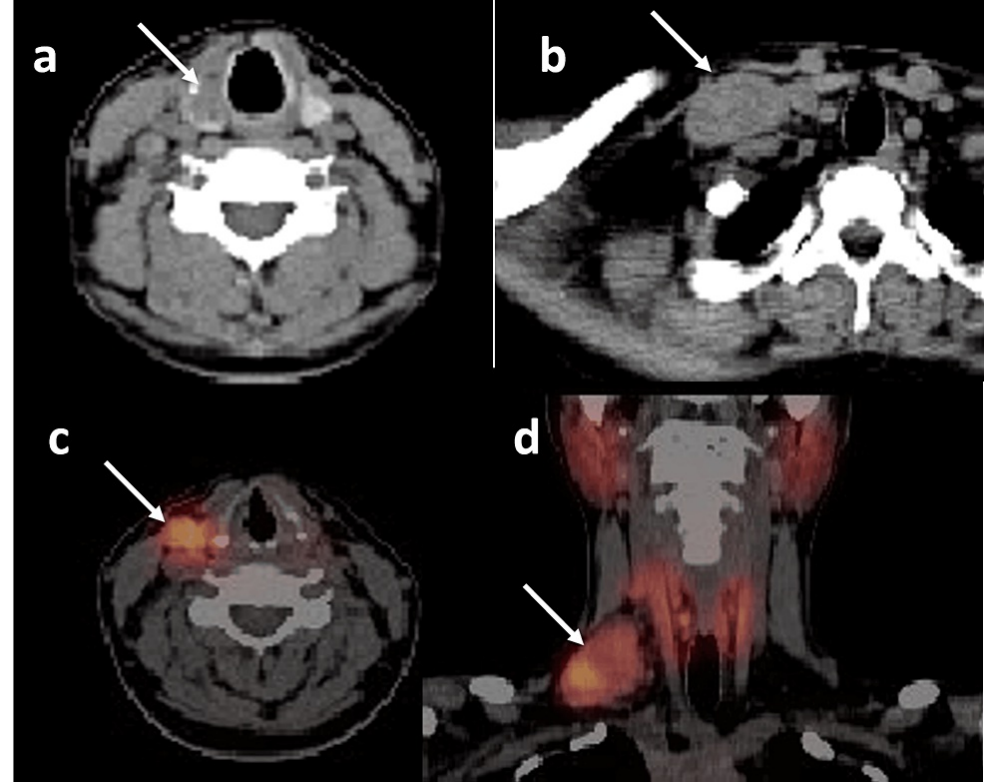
a. Axial CT images from the SPECT/CT study following thyroid uptake scan showing right thyroid lobe hypodense nodule (arrow). b: Axial CT images from the SPECT/CT showing right supraclavicular soft tissue lesion (arrow). c: Axial fused SPECT/CT image showing right level III lesion with uptake. d: Coronal SPECT/CT image showing uptake in the right supraclavicular lesion.
